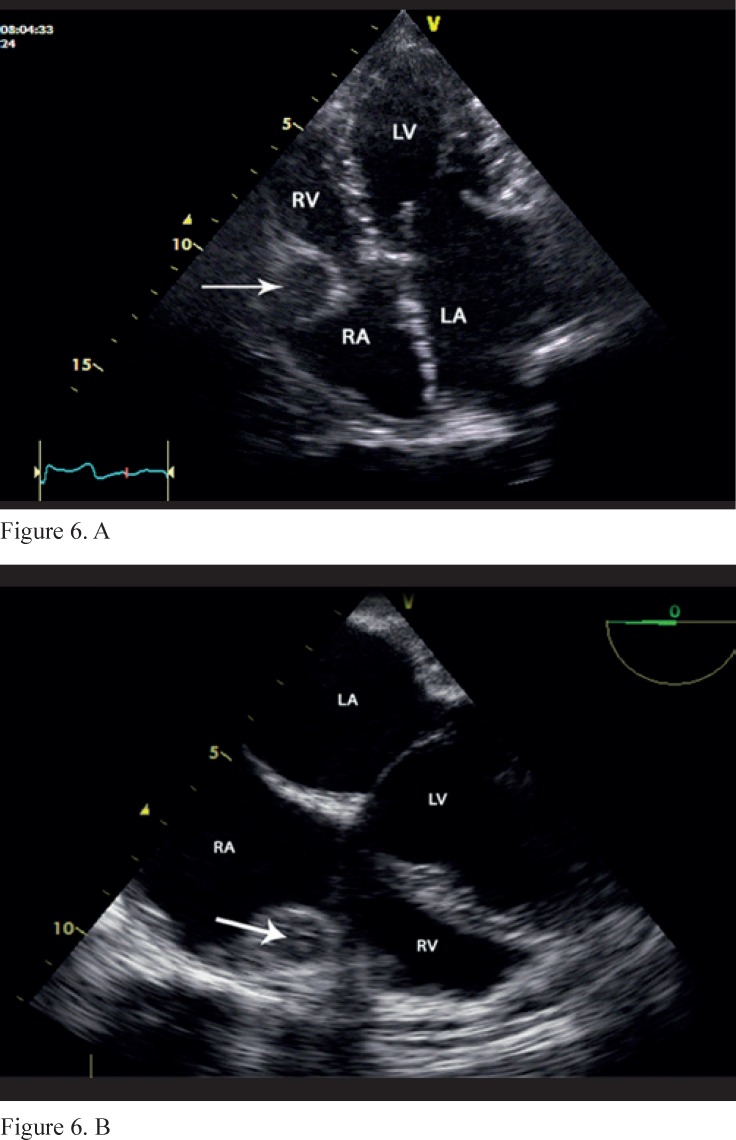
Transthoracic (A) and transesophageal (B) 2-dimensional 4-chamber view shows mass-like lesions (arrow) in the right atrioventricular groove region which partially obstruct the tricuspid valve inflow.
